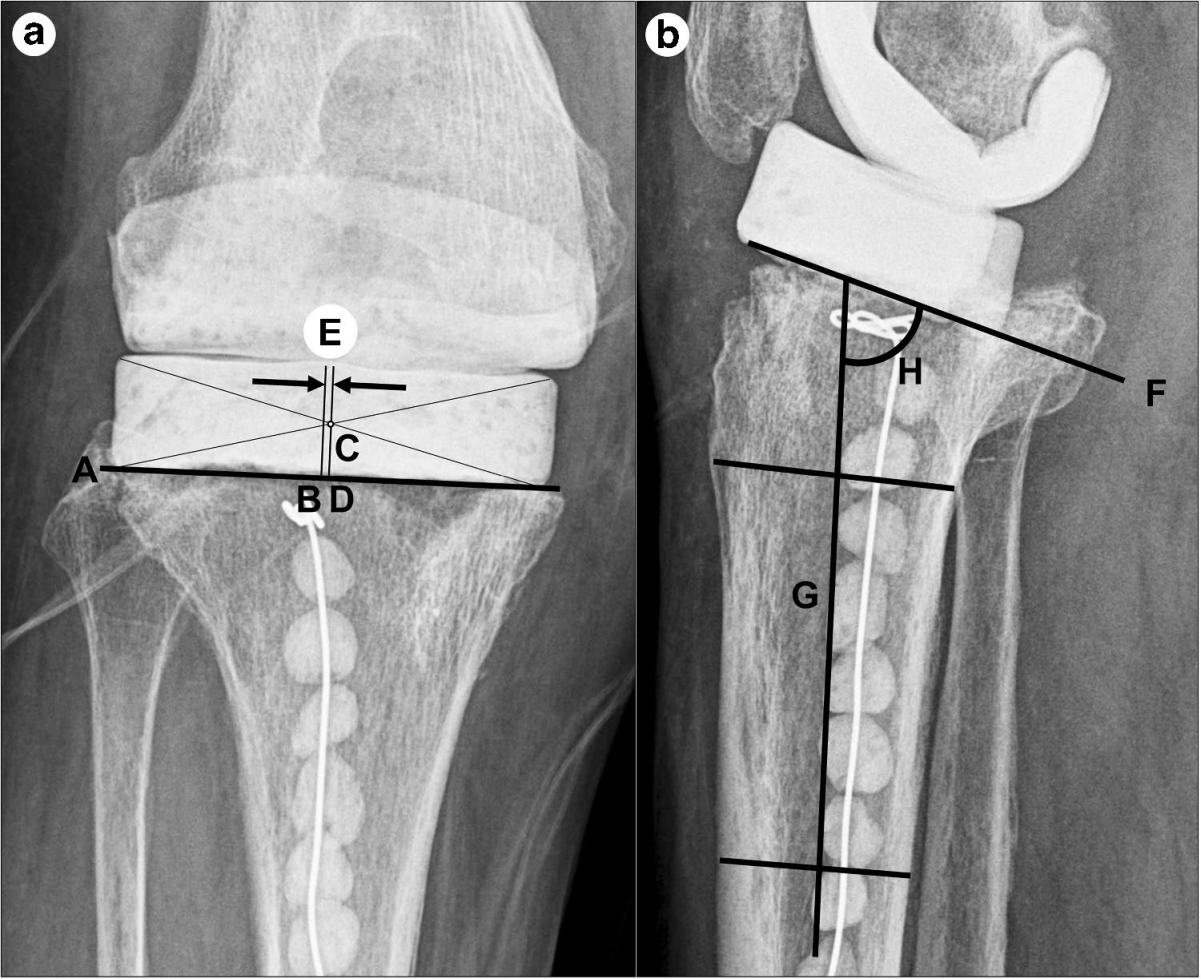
a Method of measurement of mediolateral translation of tibial cement spacer at the anteroposterior radiograph of the affected knee. b Method of measurement of tilting of tibial cement spacer at the lateral radiograph of the affected knee
